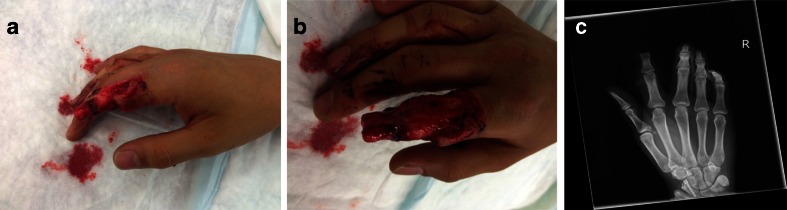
a and b Preoperative views, c Preoperative X-ray of the right hand
